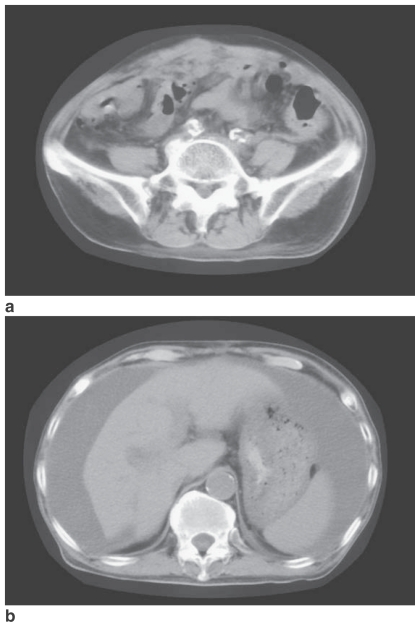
CT scans obtained after L-OHP administration. (a) The nodular masses are larger than before. (b) Ascites has increased around the spleen.
